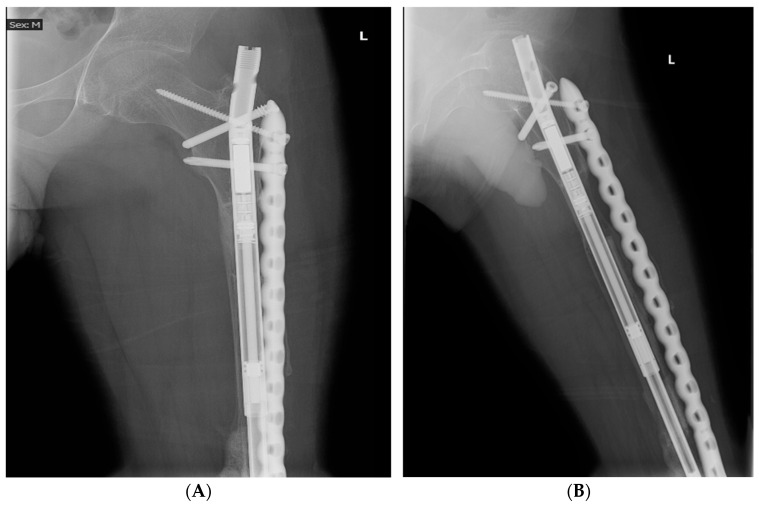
(A) Anteroposterior radiograph after initiation of the accordion maneuver showing progressive improvement in regenerate density and early corticalization, particularly along the medial cortex. (B) Lateral radiograph showing enhanced regenerate maturation and improved structural continuity following controlled cycles of compression and distraction.
